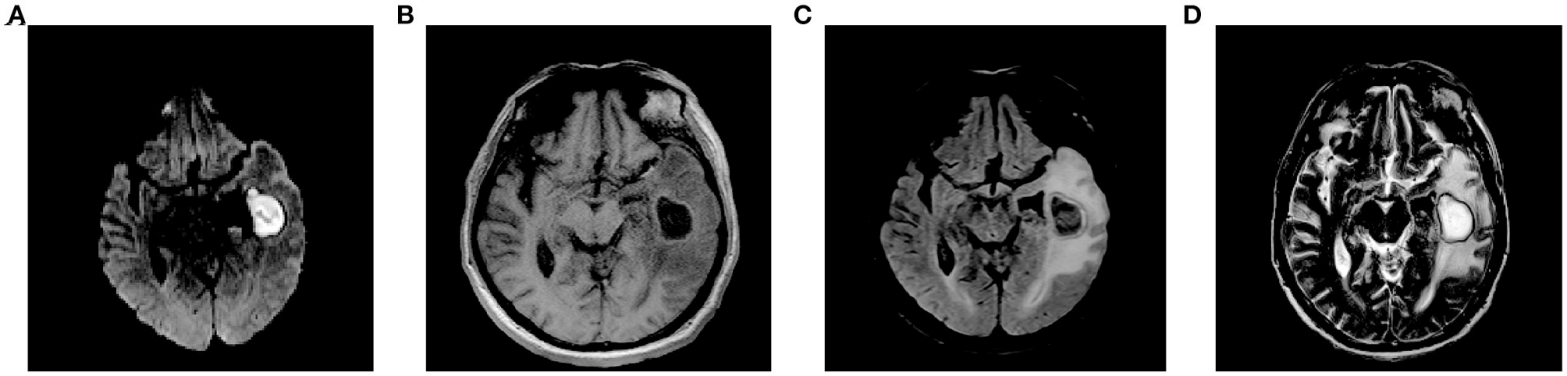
Brain MRI before mNGS. (A) DWI; (B) T1WI; (C) T2-FLAIR; (D) T2WI. MRI, magnetic resonance imaging; mNGS, metagenomic next-generation sequencing; DWI, diffusion-weighted imaging; T1WI, T1-weighted imaging; FLAIR, fluid-attenuated inversion recovery; T2WI, T2-weighted imaging.
